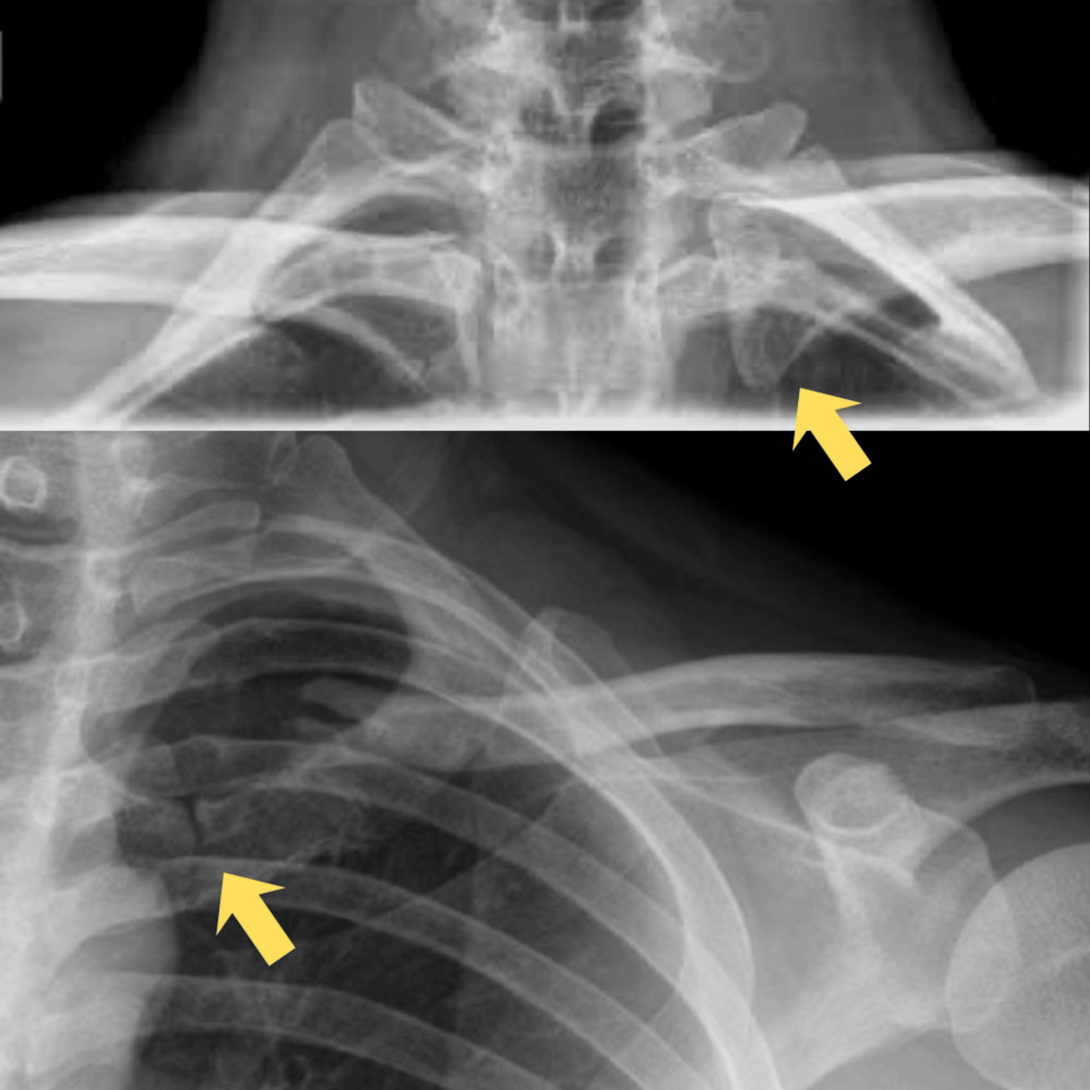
The image shows a serendipity view (above) and an anteroposterior chest X-ray view (below). Yellow arrows show an anteriorly subluxated sternoclavicular joint.
